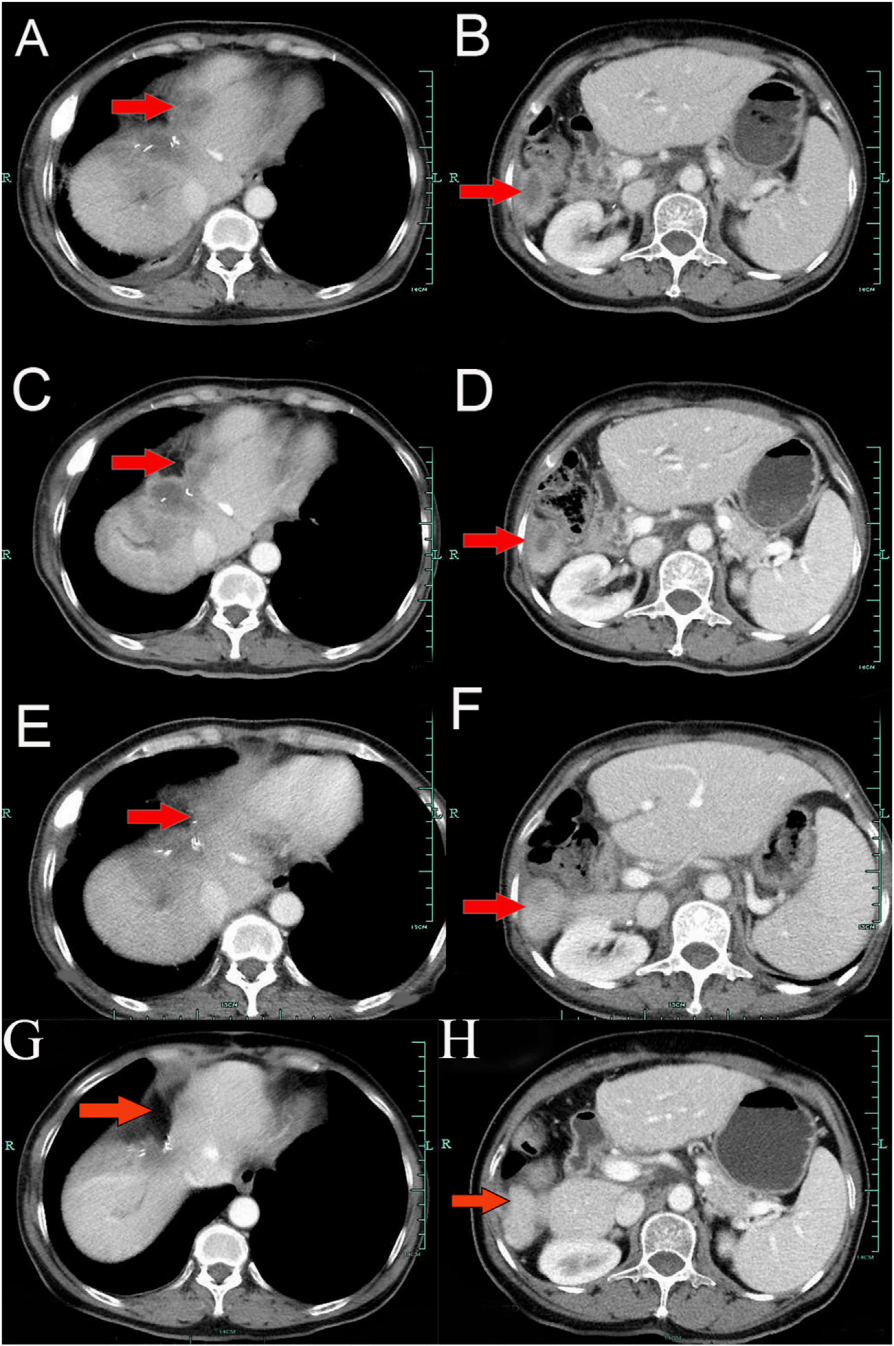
Variations of occupation on Liver areas by CT images during the treatment (red arrowheads). (A,B), March 2024. (C,D), April 2024. (E,F), June 2024. (G,H), October 2024.
